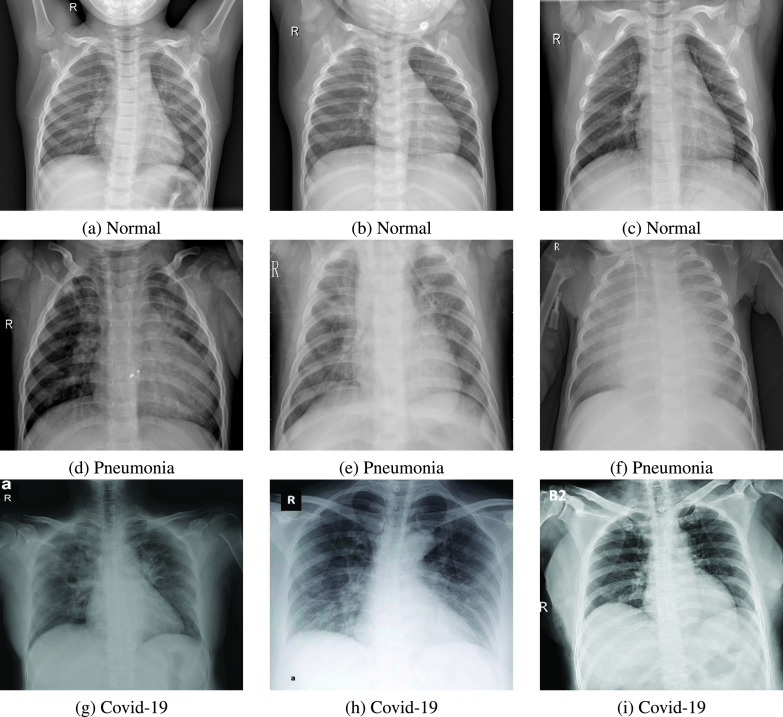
From Top to Bottom row wise: Presents normal, pneumonia and COVID-19 X-ray images.
